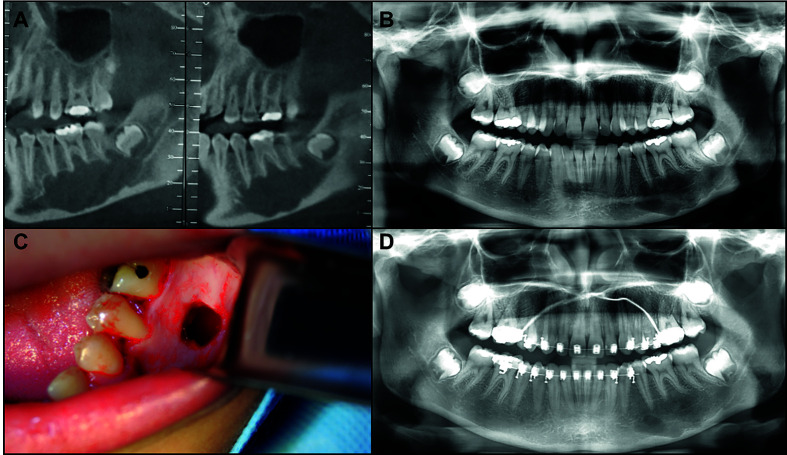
A: Parasagittal tomographic aspect of a mandibular SBC; B: Panoramic radiograph showing SBC lesion in left posterior portion of mandible involving second premolar, first and second molar. C: Surgical exploration of the lesion; D: Pos surgical radiograph evidencing bone formation.
